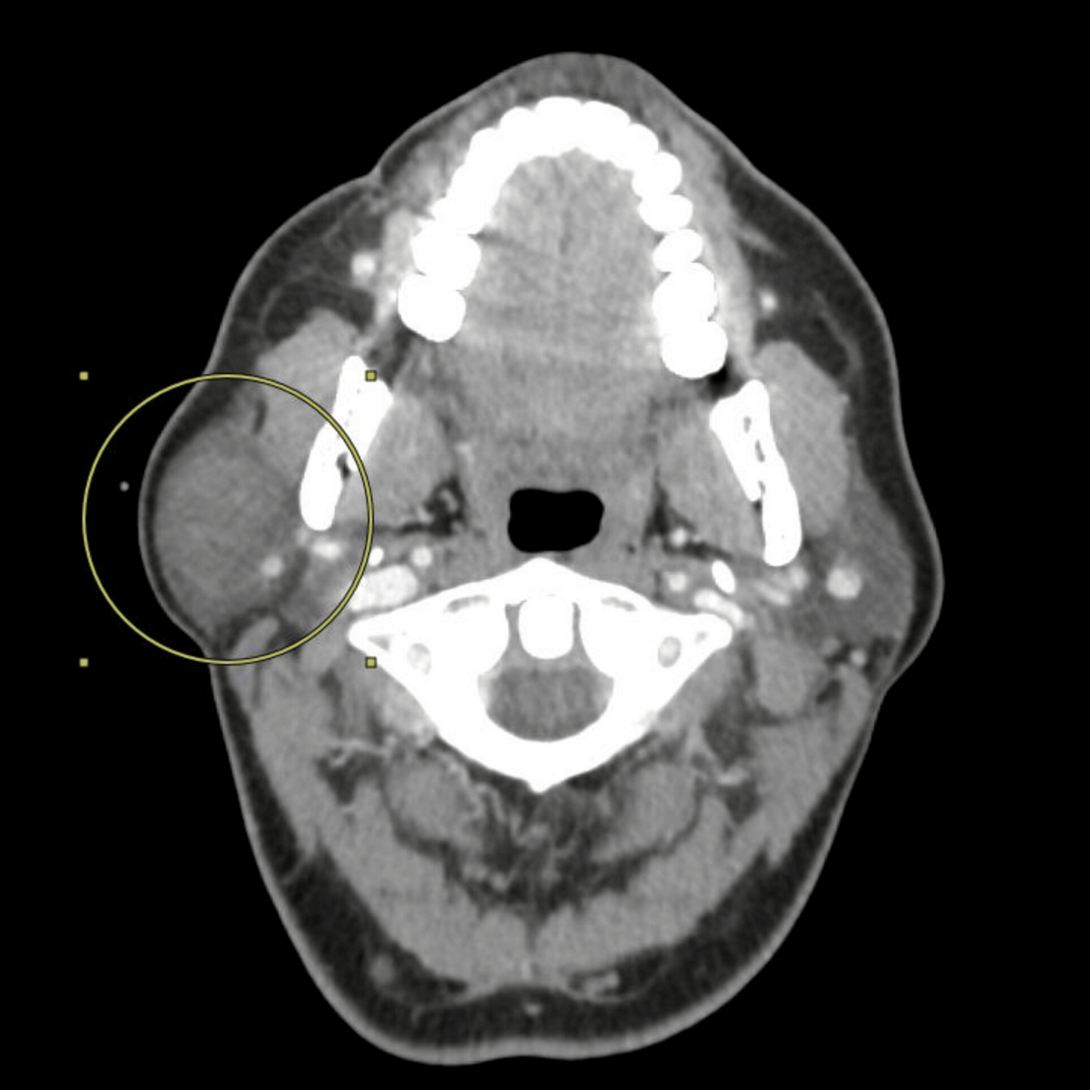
Axial CT of the neck with contrast revealing multiloculated lesion of the right parotid gland with heterogenous cystic and solid appearance. CT: computed tomography
